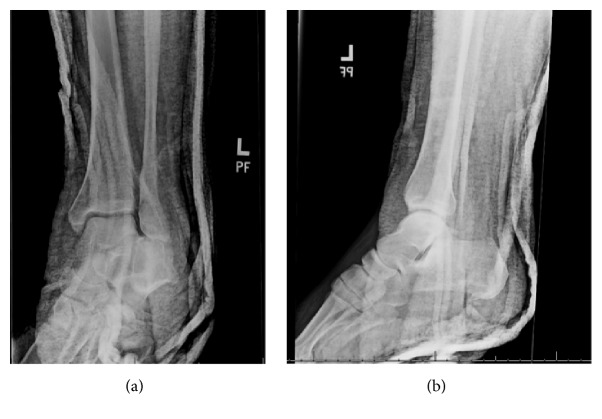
Postreduction radiographs demonstrating congruent reduction of the subtalar joint.
